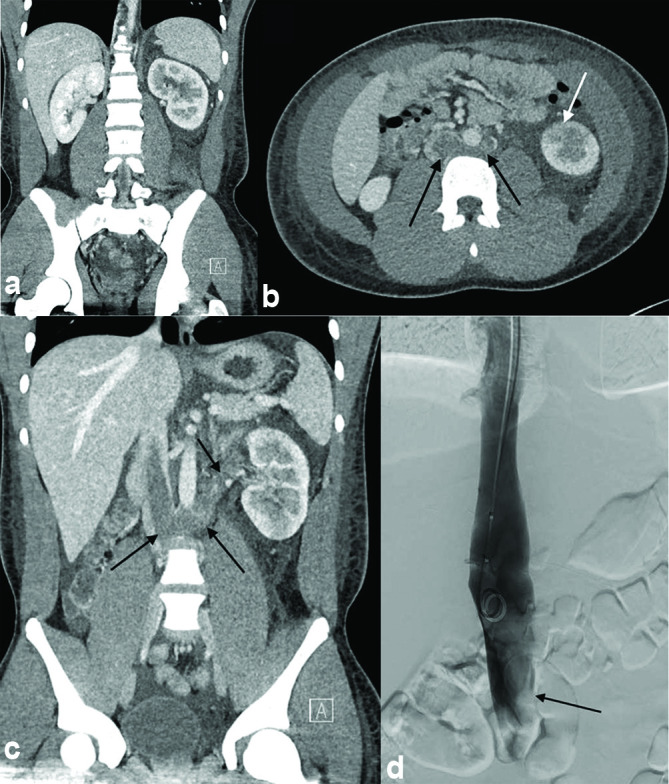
Multiplanar intravenous contrast-enhanced CT of the abdomen and pelvis demonstrates enlargement of the left kidney, moderate left-sided perinephric free fluid (a) and a delayed left nephrogram (white arrow—b). Acute thrombus was noted in a tortuous circumaortic left renal vein, which extends into the inferior vena cava and measures up to 12.0 cm in craniocaudal dimension (black arrows—b, c). Subsequent anteroposterior projection cavogram demonstrates inferior vena cava thrombus, which extends downstream to the approximate level of the right renal vein (black arrow—d).
